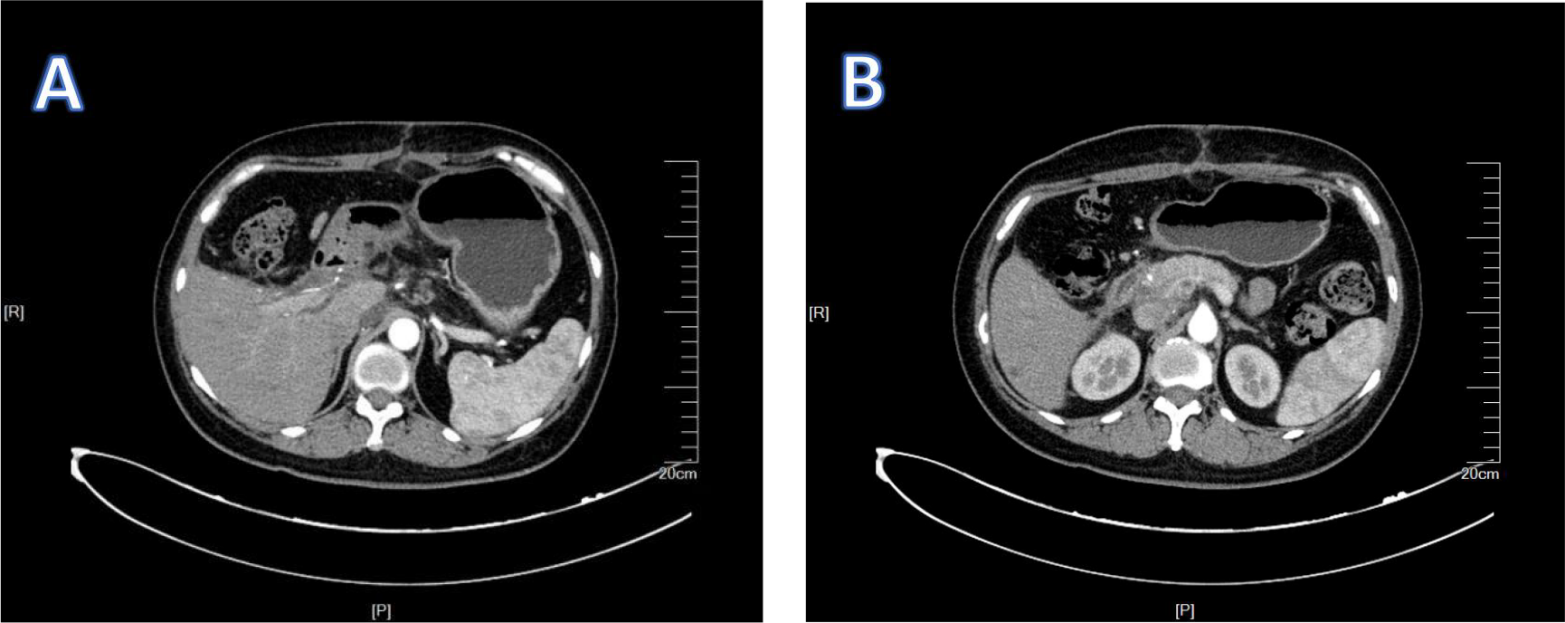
1Postoperative examination of patient 1. (A, B) show the CT examination results of patient 1 after the operation.
